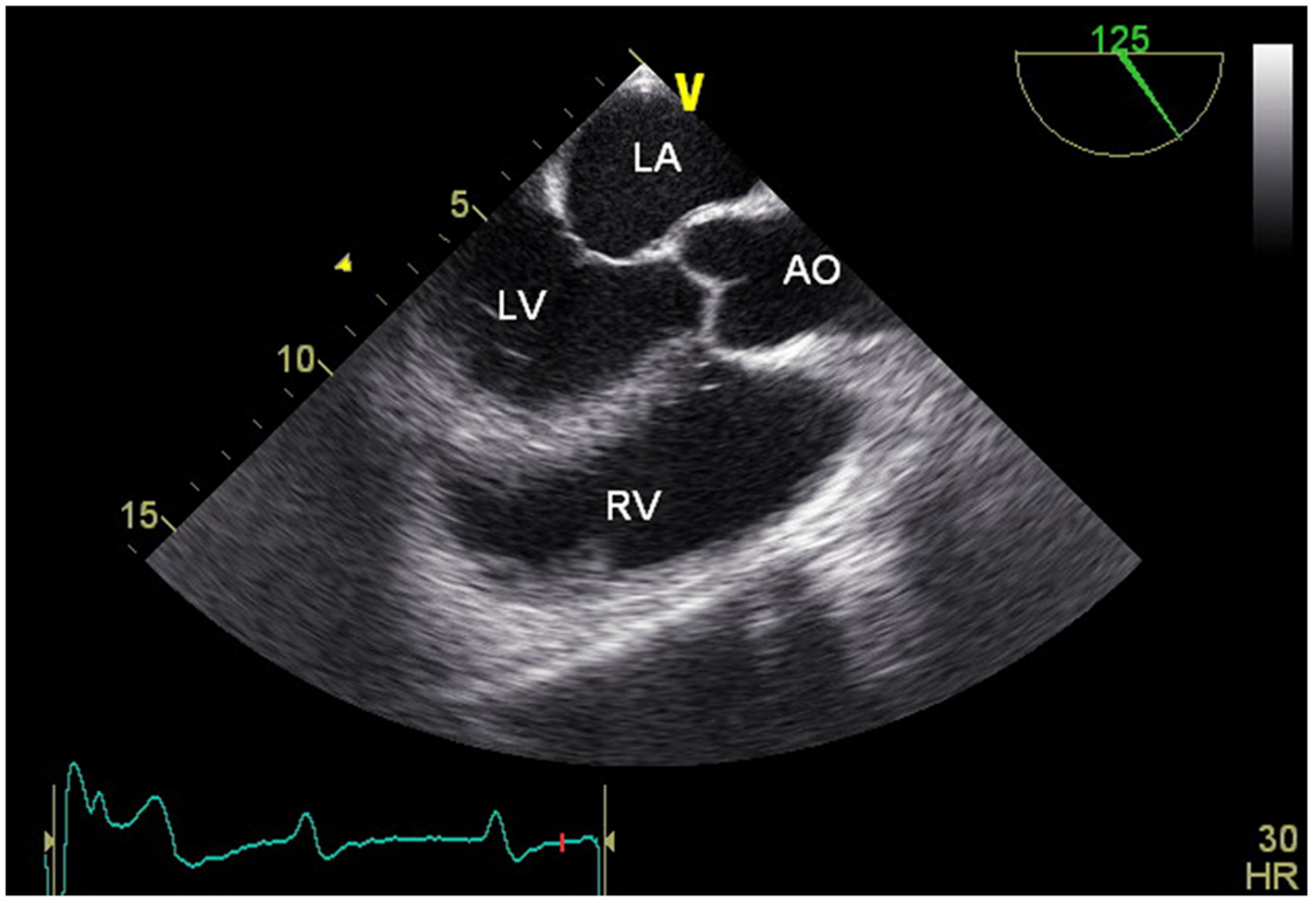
Four weeks after treatment with penicillin intravenous transesophageal echocardiography shows no vegetations. LA: left atrium, LV: left ventricle, RV: right ventricle, AO: aorta.
